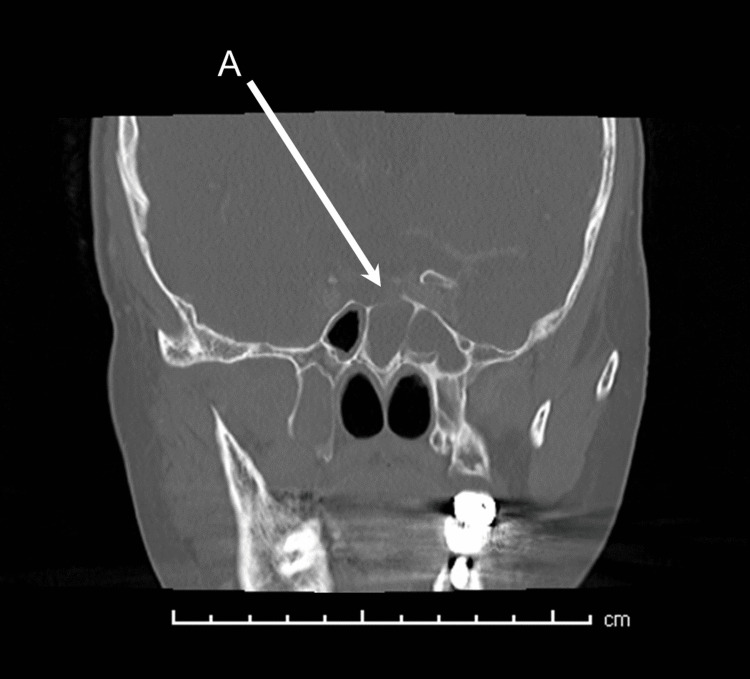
CT image showing opacified sphenoid sinus with accessory bony septations and a defect between the left side and the sella turcica (A)
